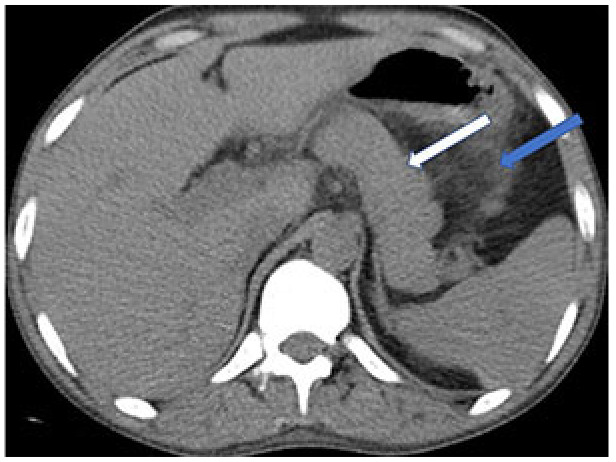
A 30-year-old male COVID-19 patient presenting with abdominal pain. CT scan shows volume increase in the pancreas (white arrow) and increased density in the surrounding fatty tissue (blue arrow), consistent with pancreatitis.
